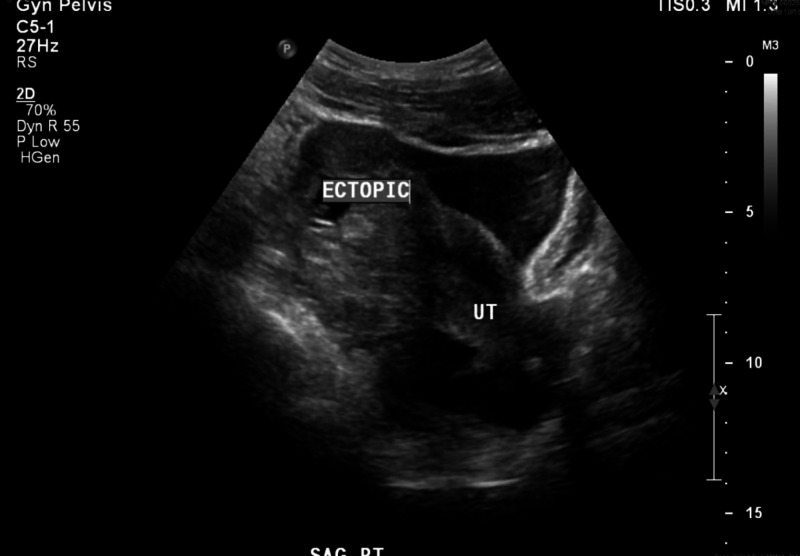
Day 10 ultrasonography (US) of cornual ectopic pregnancy rupture with hemoperitoneum
